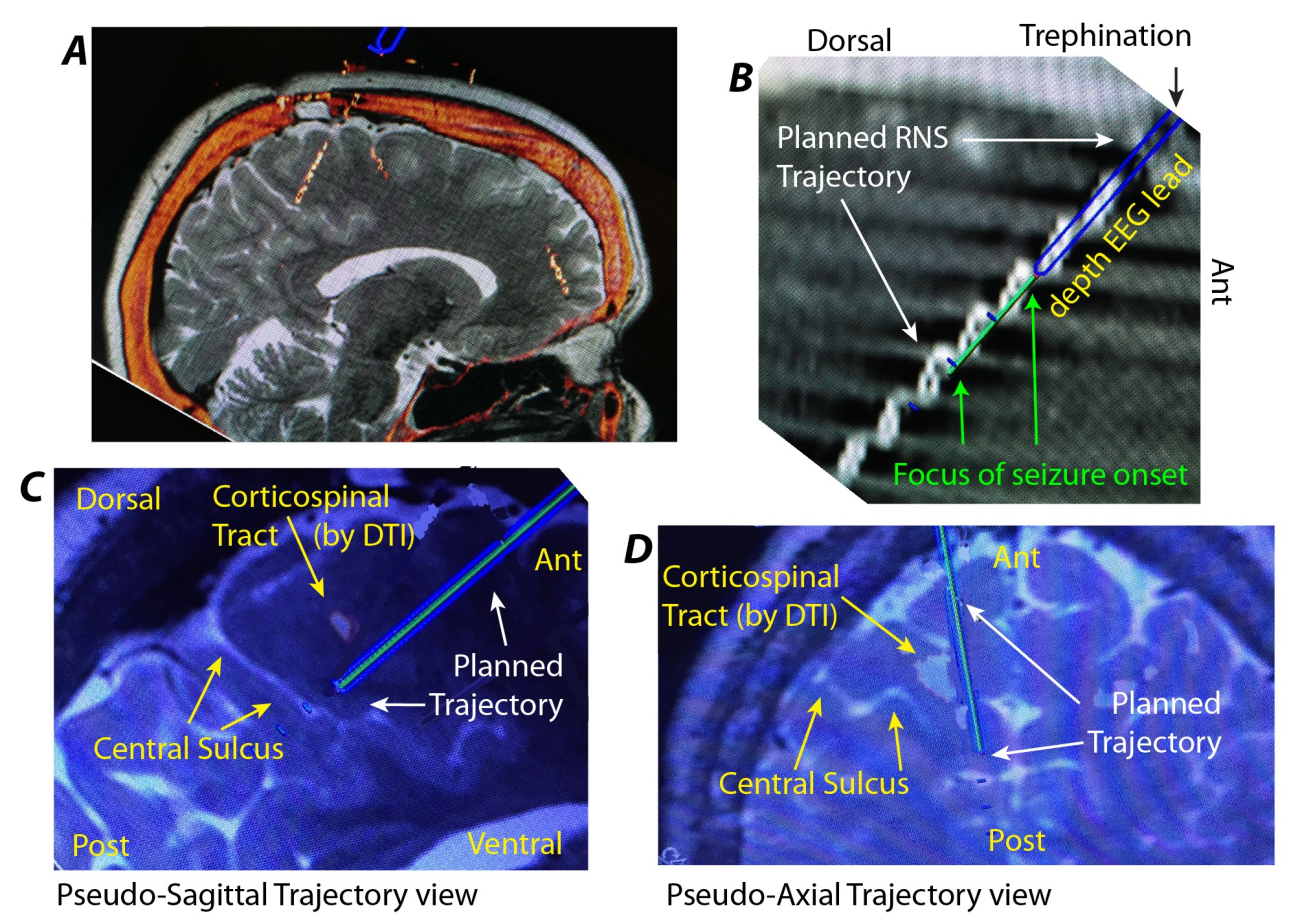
Stereotactic Planning *(A) *Sagittal slice through fused CT & MRI, showing depth EEG electrodes in situ. *(B)* The trajectory for the RNS electrodes is determined by planning a trajectory that passes directly through the trephination and terminates at the depth EEG site of seizure focus identification. *(C)* Anatomical MRIs as well as specialized scans such at diffusion tractography are fused to the base-CT, and allow direct comparison with planned trajectory. A pseudo-sagittal trajectory view is shown.* (D) *As in (C), but for a pseudo-axial trajectory view.
